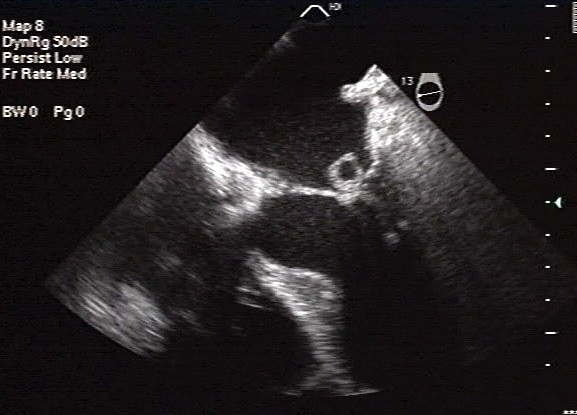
Transesophageal echocardiogram. A rounded mass, 1 cm in diameter, attached to the auricular side of the anterior mitral valve leaflet, compatible with vegetation and abscess, is observed. A smaller mass, 0.5 cm in diameter, is observed on top of the abovementioned mass, causing mild mitral failure.
